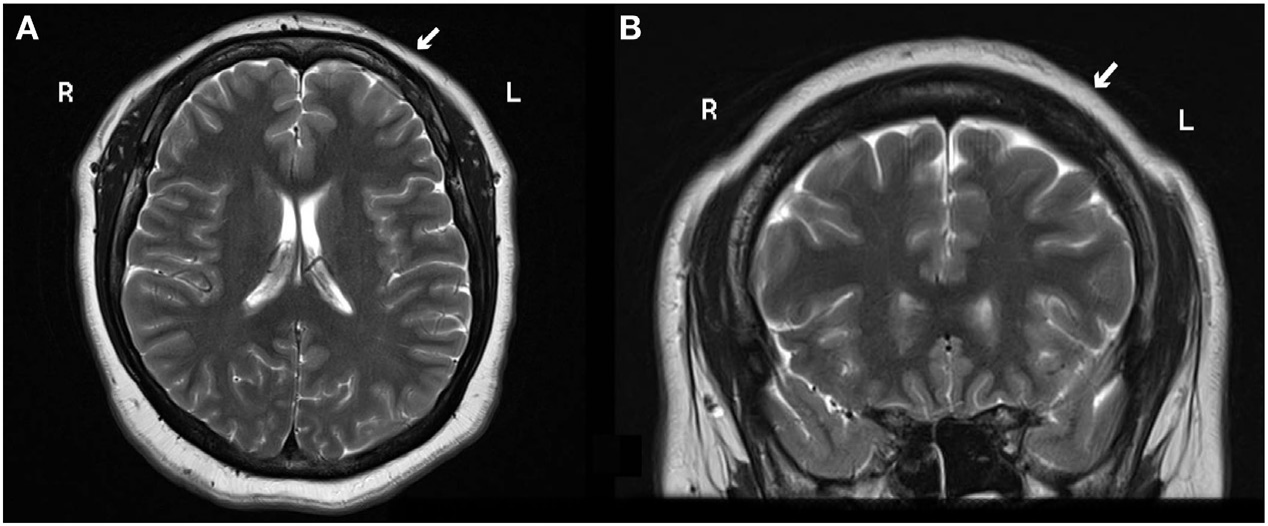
(A) Axial and (B) coronal 3 T MRI head scan shows blurry cortical gray and white matter on the left frontal lobe suggestive of focal cortical dysplasia (white arrow). Abbreviations: R, right; L, left.
